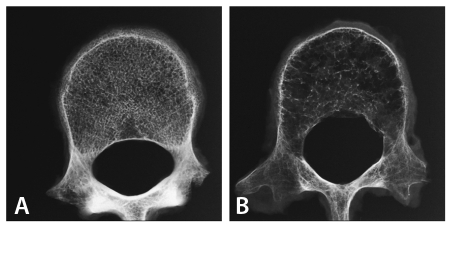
Radiographic images of lumbar vertebrae from (a) normal and (b) osteoporotic patients.
